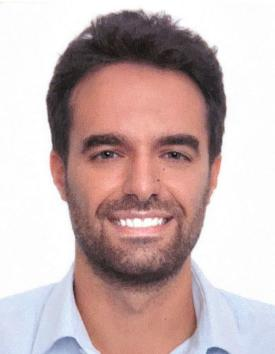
Dr Edoardo Sciatti works at the Heart Failure Clinic of ASST Papa Giovanni XXIII in Bergamo, Italy, and is involved in several international trials about HFrEF and HFpEF therapy. His main interests beyond heart failure are valvular heart diseases, echocardiography in interventional cardiology, and cardiovascular complications of pregnancy.
